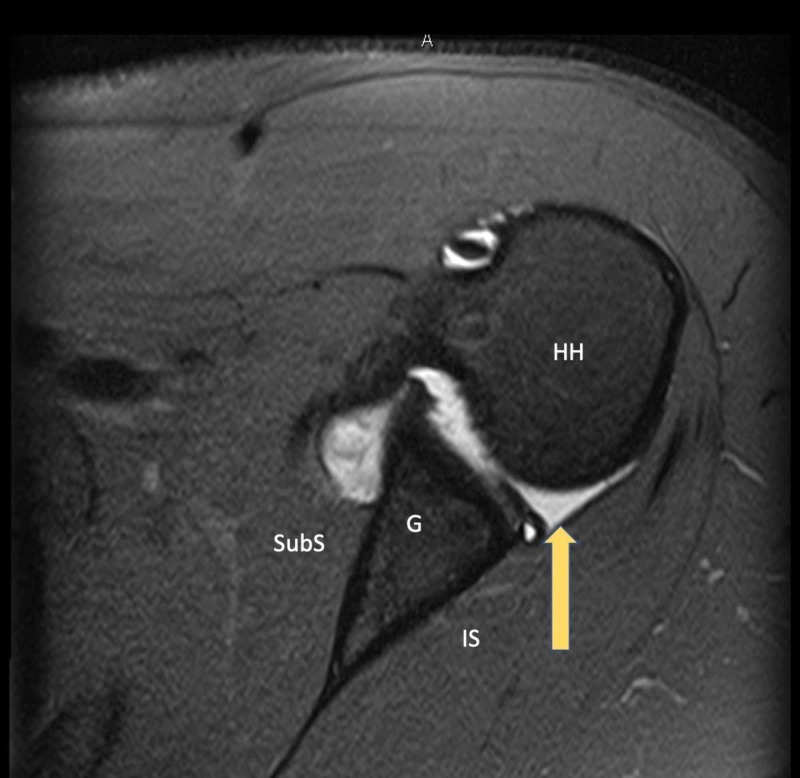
Magnetic Resonance Imaging (MRI) Demonstrating a Posterior Labral Tear Axial plane T2 fat-saturated image demonstrating a displaced posterior labral tear in a symptomatic athlete with a batter’s shoulder injury. Arrow - Posterior labral tear HH - Humeral head; G - Glenoid; SubS - Subscapularis; IS - Infraspinatus
